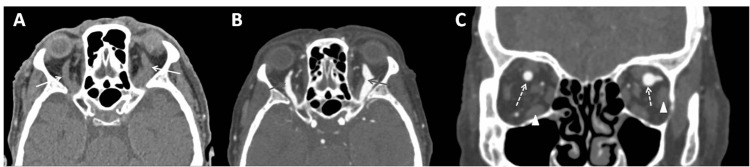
Computed tomography scan of the head (A) Axial computed tomography scan of head and orbit performed urgently shows bilaterally dilated superior ophthalmic veins (white arrows). (B) Axial computed tomography angiography confirms dilated superior ophthalmic veins (arrows) consistent with a diagnosis of direct carotid-cavernous fistula. (C) Coronal computed tomography angiography shows a mild enlargement of extraocular muscles (arrowhead) and dilated superior ophthalmic veins (dotted arrows).
